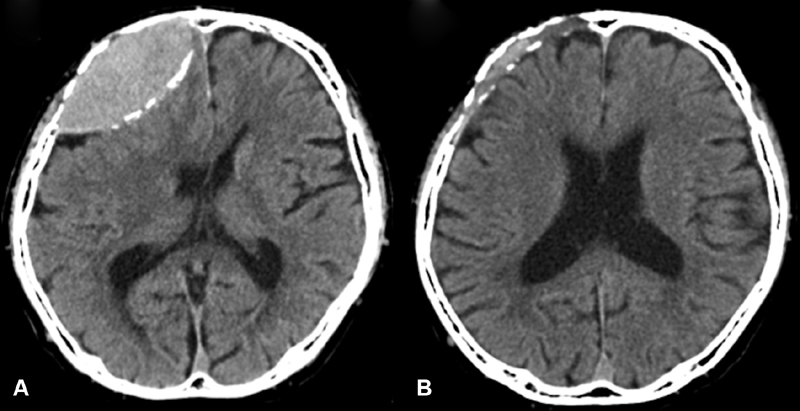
Computed tomography scan on admission (
